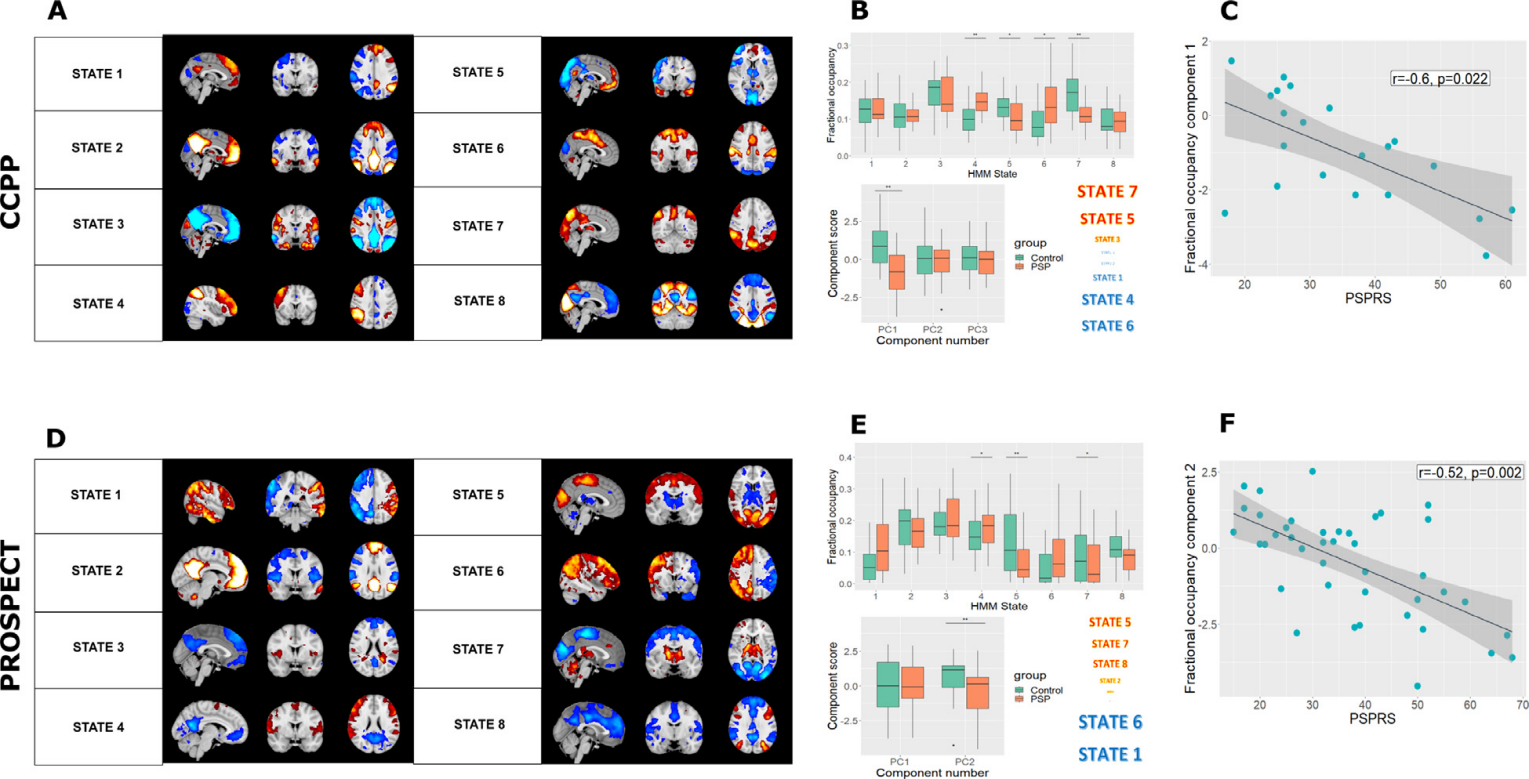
Network dynamics in PSP vs controls. (A) and (D) Mean activation maps for the 8 inferred brain states from hidden Markov modelling for the two cohorts. (B) and (E) Altered fractional occupancy rates in PSP for the two cohorts. Results are shown both by differences in states computed within a general linear model with a single permutation test and family-wise error correction, and in a principal component analysis of fractional occupancy rates. In both cohorts, participants with PSP spent less time in states with subcortical and posterior activation, and more time in frontoparietal states. Colours of state names indicate direction of principal component loading, and font size scales with their magnitude. (C) and (F) The component that differed between PSP and controls correlated with PSP rating scale among patients (CCPP r=-0.6, *p*=0.02; PROSPECT r=-0.52, *p*=0.002).
